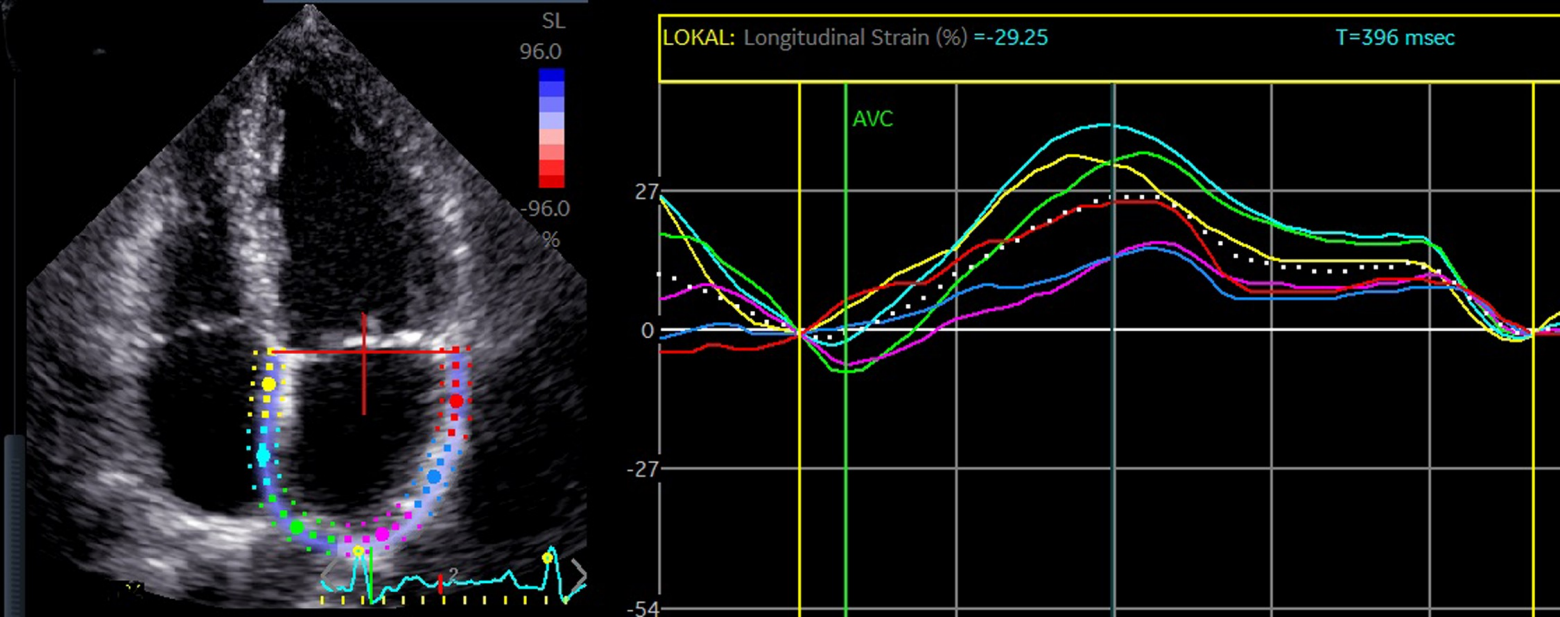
Two-dimensional left atrial (LA) reservoir strain from apical 4-chamber view. The current proband shows a LA strain function of 26%.
